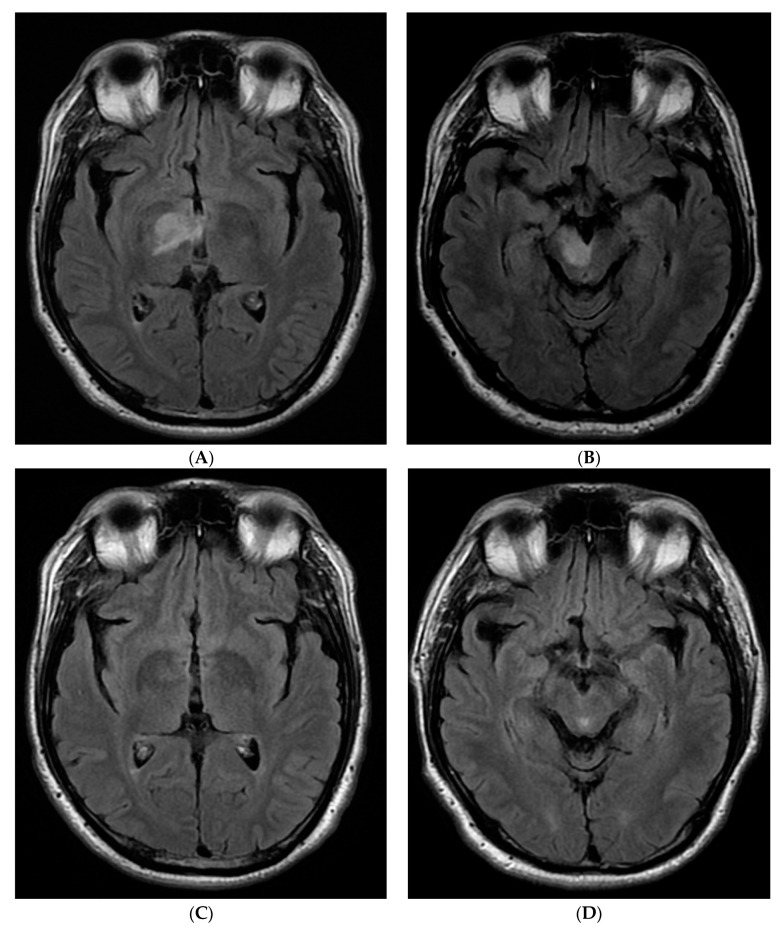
Selected magnetic resonance imaging (MRI) T2/fluid-attenuated inversion recovery (FLAIR) imaging from initial (A,B from Jan 2017) and last (C,D from May 2017) MRI.
